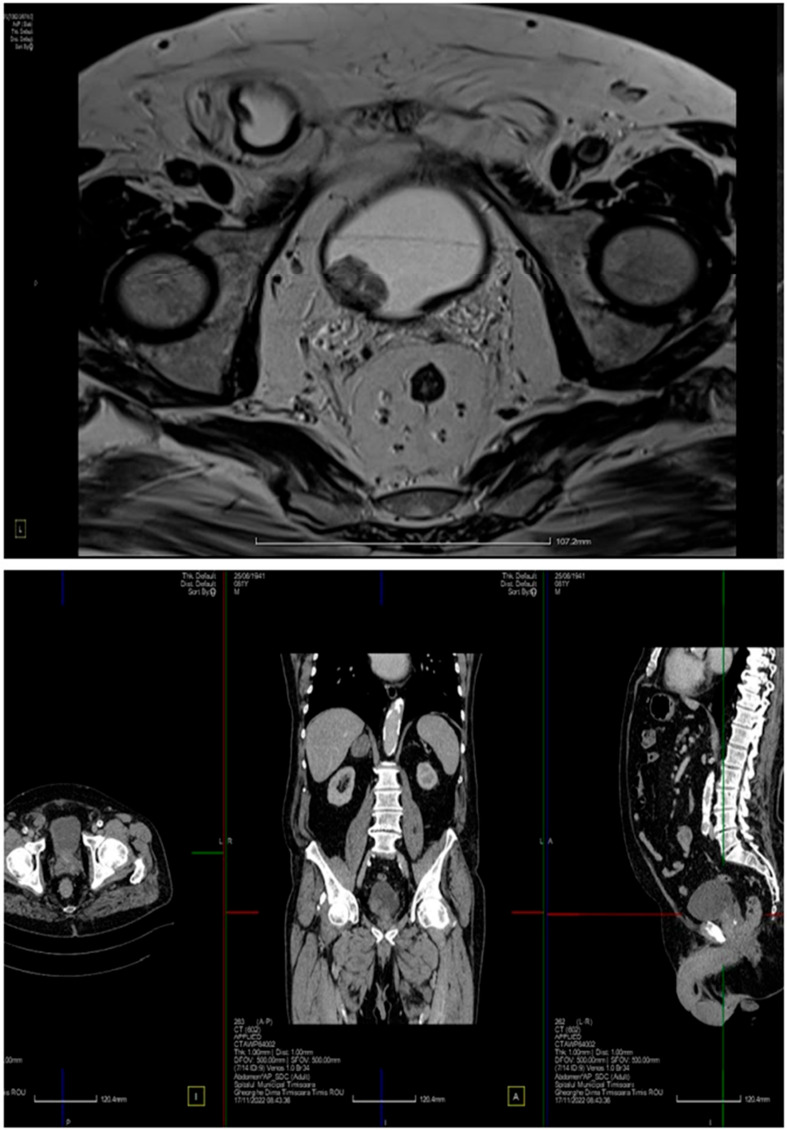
MRI imaging (upper), and abdominal and pelvic CT (lower).
